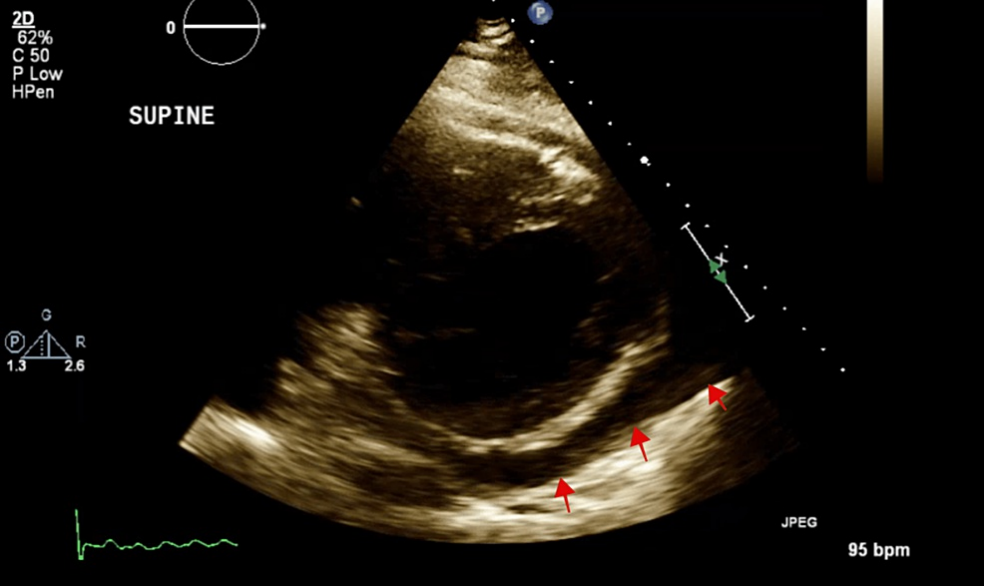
A 2D-Echocardiography showing moderate pericardial effusion (red arrows).
